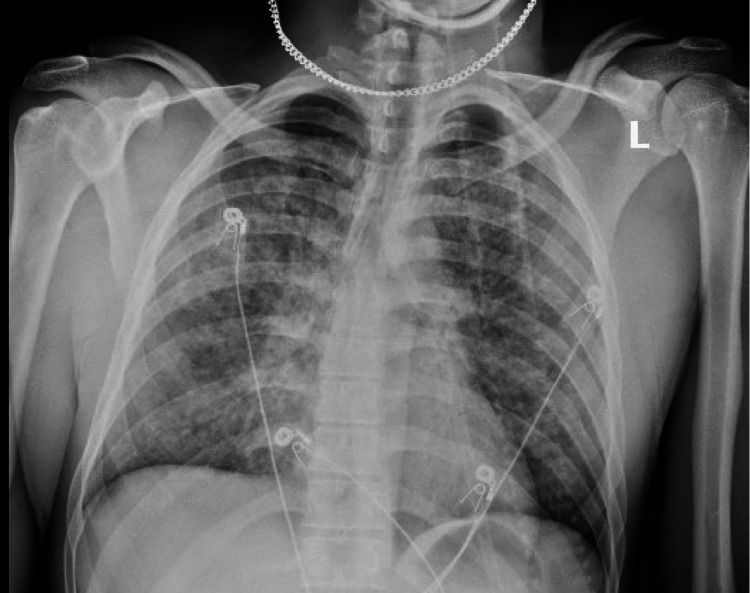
Chest X-ray on day one with diffuse bilateral infiltrates.
